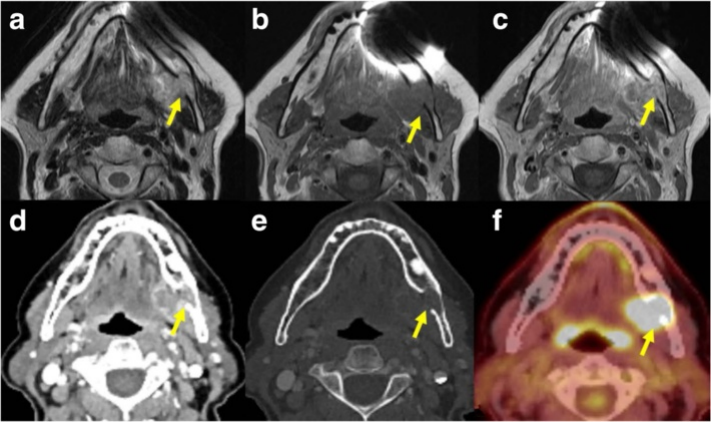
Patient with carcinoma ex-pleomorphic adenoma of the submandibular gland on the left with invasion of the mandible. In the T2-weighted MR image a the lesion is heterogenously hyperintense to muscle and there is loss of the cortical hypointense line indicating bone invasion (arrow). In the T1-weighted image b the lesion is hypointense to the muscle and there is replacement of the normal high signal marrow fat by the tumour. The T1-weighted image c after injection of gadolinium without fat saturation shows some enhancement of the lesion. Contrast enhanced CT scan at the same level d shows the heterogenously enhancing mass which extends into the mandible. The cortical bone erosion (arrow) is seen better in the bone window setting e. The PET-CT scan f at the same level showing the increased glucose uptake by the tumour with extension into the mandible
